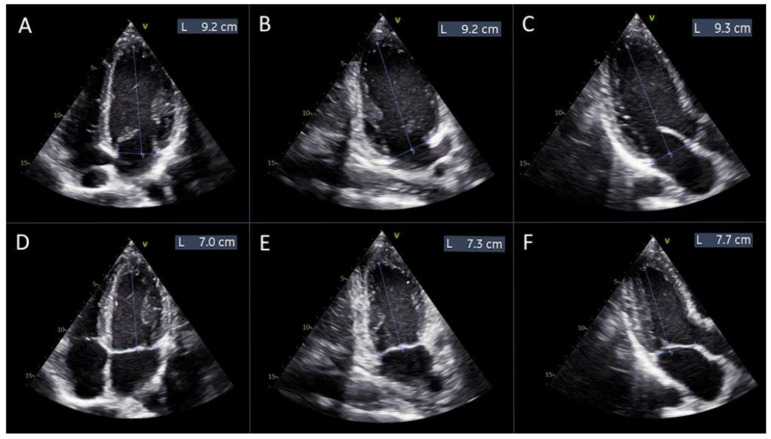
Measurements of left ventricular length for manual global longitudinal strain calculation. Panels (A–C) display the measurements of the left ventricular length obtained from the apical 4-chamber, 2-chamber, and 3-chamber views, respectively, at diastole. Panels (D–F) show the corresponding measurements from the same apical views at systole.
